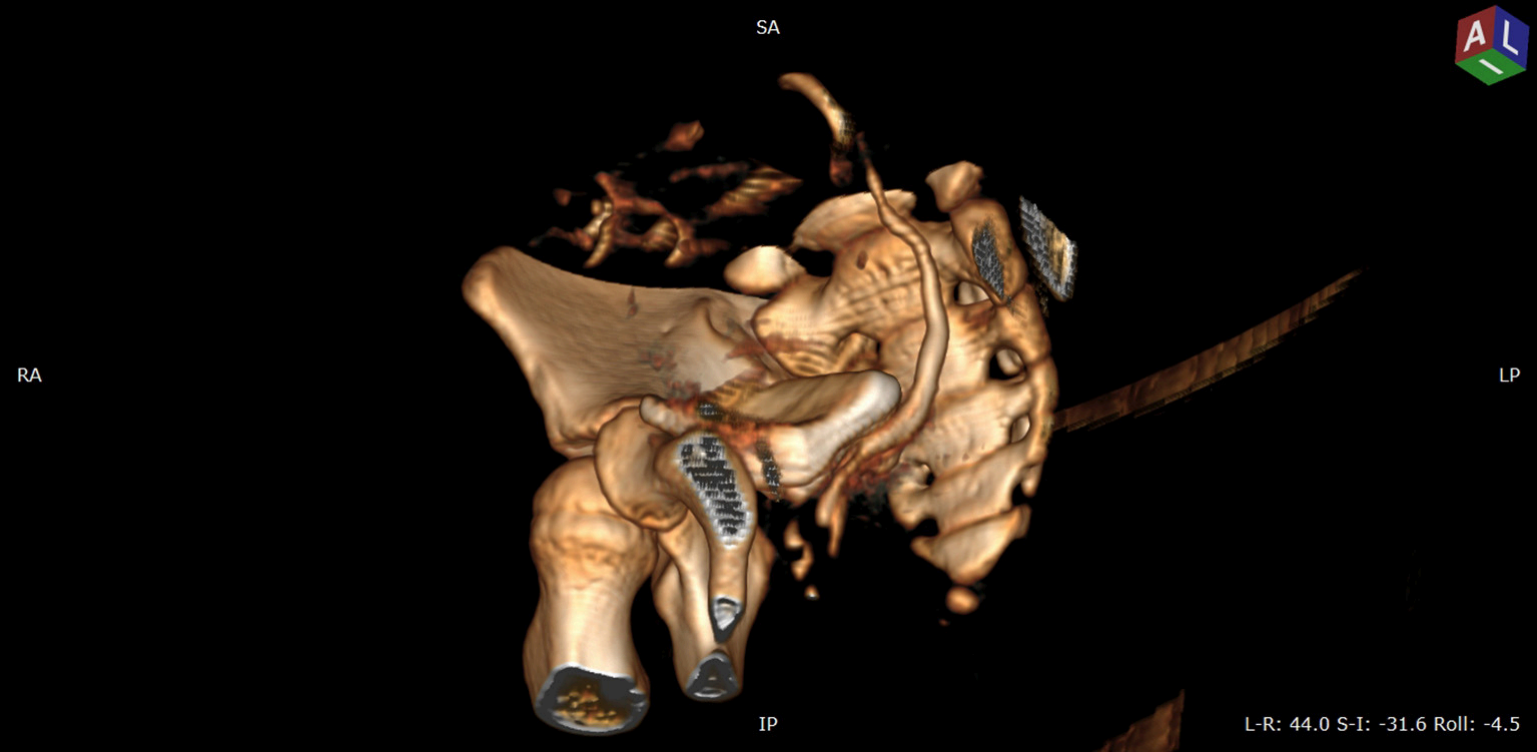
VRT (volume rendering technique) CT image, urographic sequence—oblique anterior view after removing left coxal bone—paravesical path of left ureter that insert into vagina.
